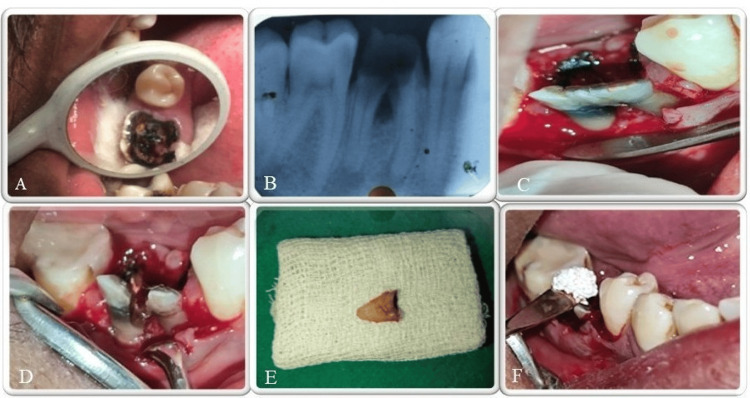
Pre- and intraoperative images A) Pre-operative clinical picture; B) Pre-operative radiograph #46; C) Envelope flap raised; D) Sectioning of #46; E) Extracted distal root; F) Bone graft placed in the distal root socket.
